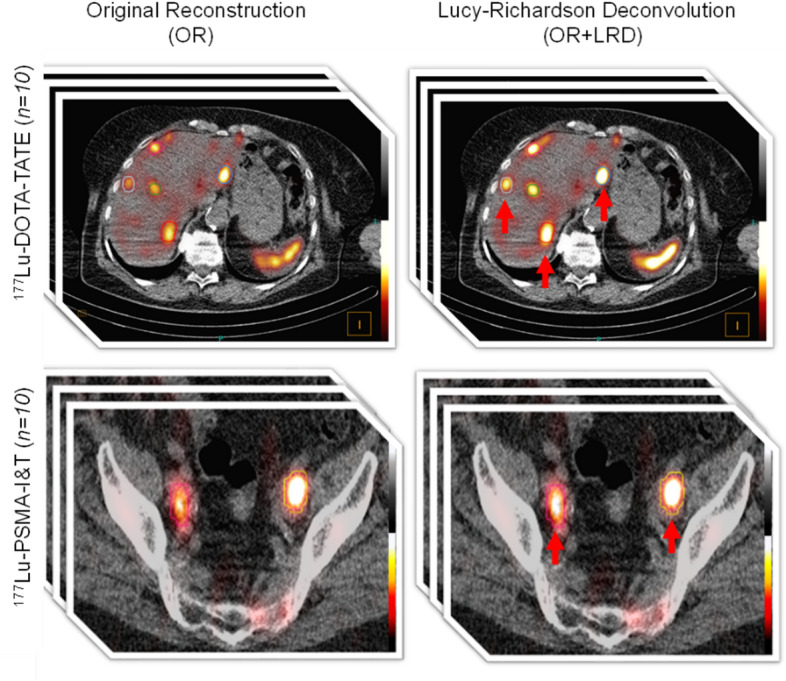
Exemplary SPECT/CT data for both patient cohorts acquired at three time points post injection with and without LRD applied. Red arrows indicate selected lesions
